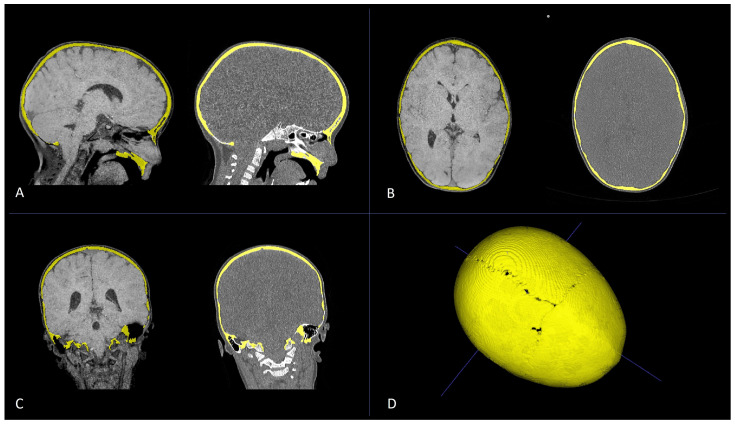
Multiplanar reconstruction (A–C) of CT scan (left) and black-bone MR image (right) of a patient diagnosed with PT for premature fusion of the metopic suture. Axial (A), sagittal (B), and coronal (C) plane reconstructions were used to obtain bone segmentation (yellow) and subsequent volumetric rendering from black-bone MR images (D).
